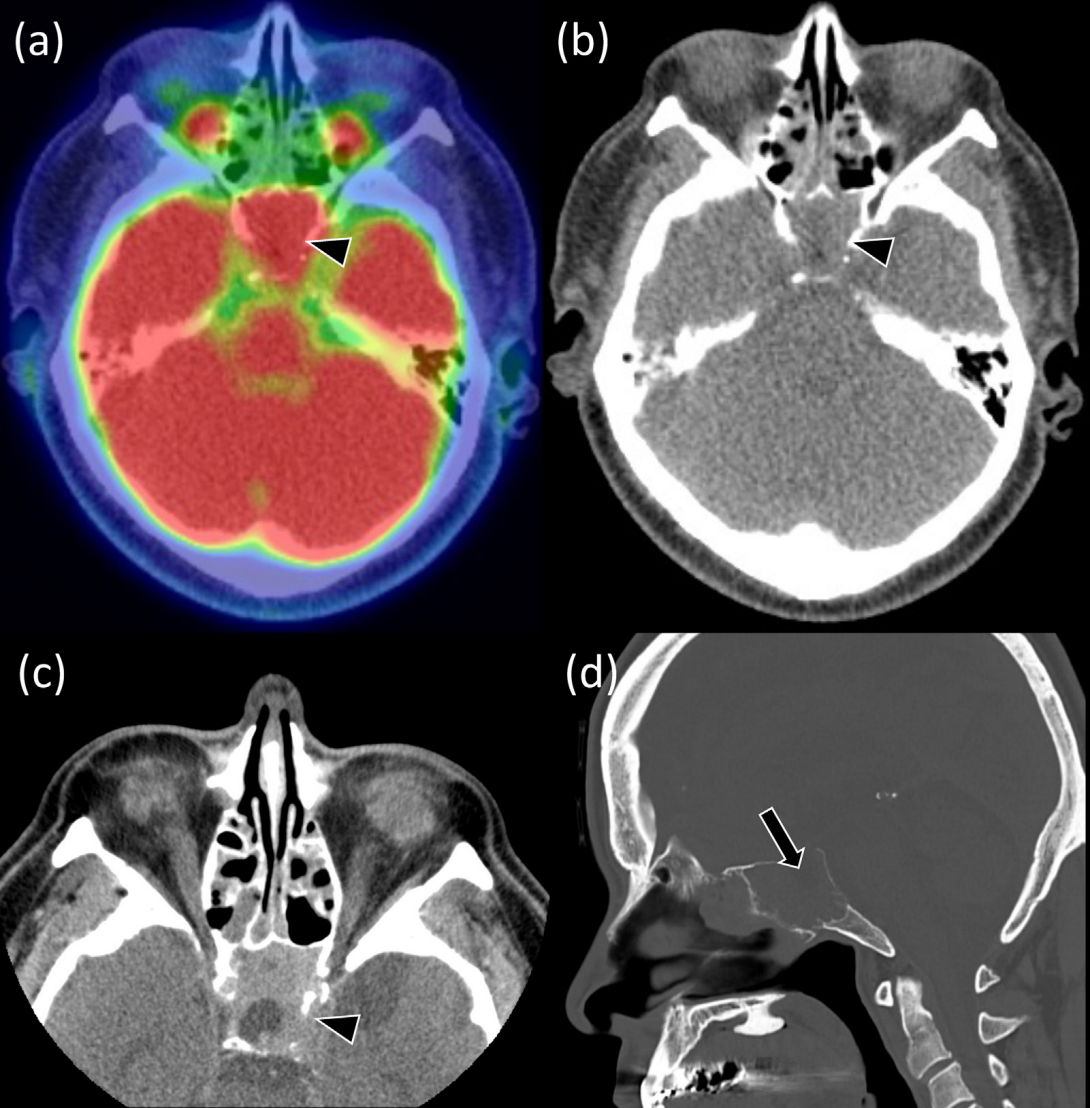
The mass in the sphenoid sinus on PET-CT and CT. 18F-FDG PET/CT shows soft density mass with a high FDG accumulation (SUV max, 16.1) filling the sphenoid sinus (A, B, arrowheads). The mass shows heterogeneous contrast enhancement on postcontrast CT (C, arrowhead). There are lytic changes in the clivus with a sclerotic rim, and the bone wall at the base of the sella turcica is hardly identified (D, arrow).
